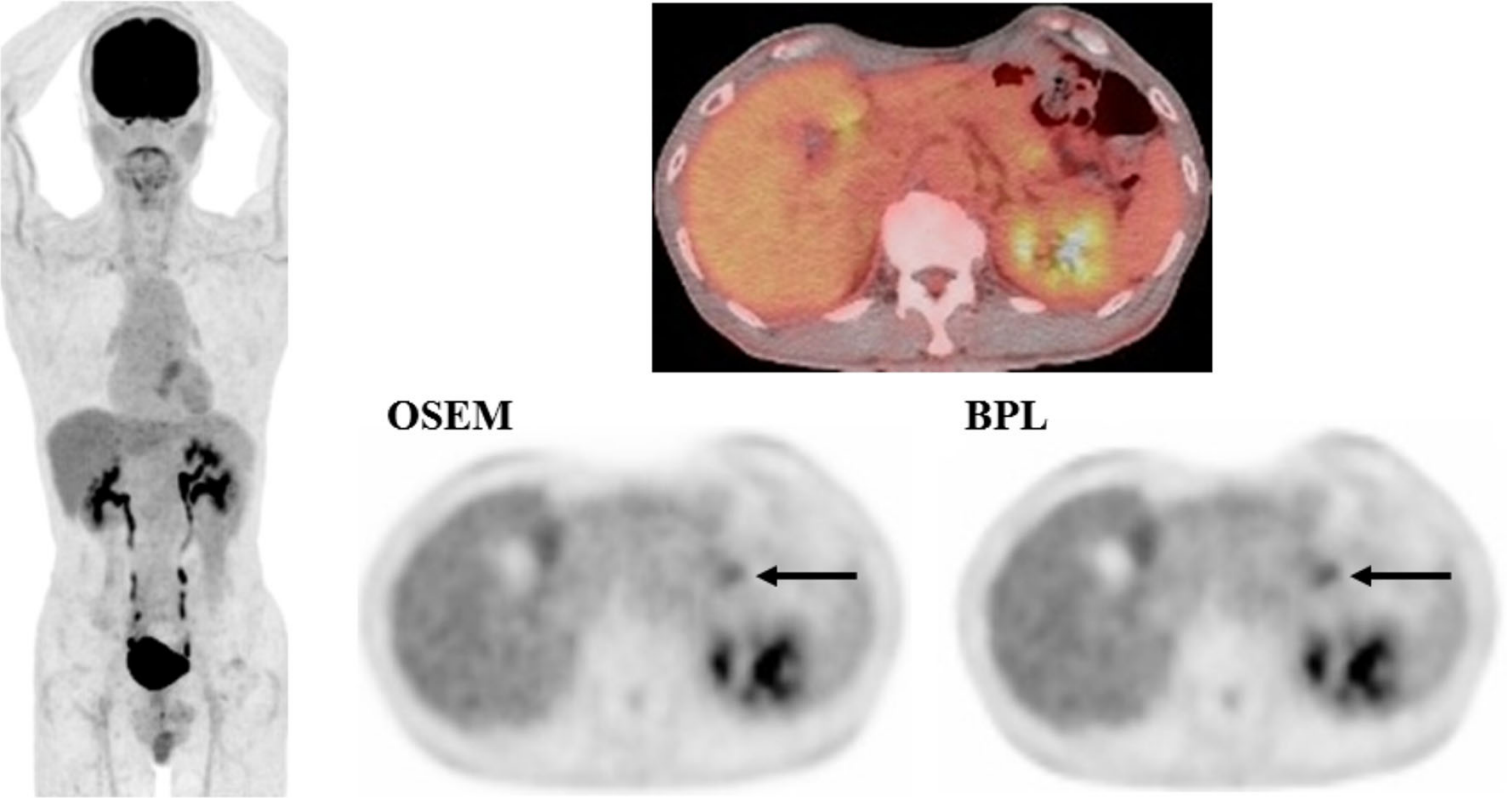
A case with pancreatic cancer (small lesion). FDG uptake corresponding to the pancreatic cancer lesion (arrow) was observed more clearly in BPL than OSEM image (right, lower). SUVmax was higher in BPL (2.9) than in OSEM image (2.7). (Left: maximum intensity projection image of FDG PET; right upper: fused FDG PET/CT image; right lower: FDG PET images reconstructed with OSEM and BPL algorithms.).
